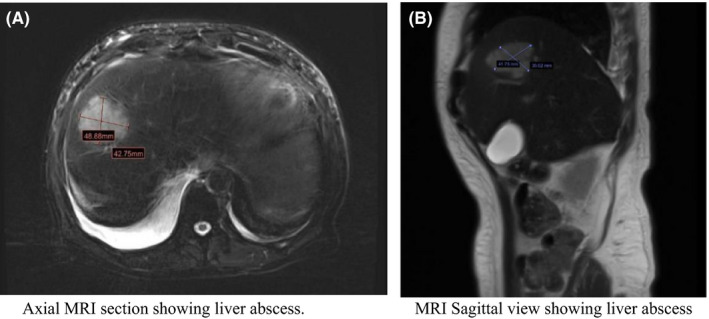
(A) Axial MRI section showing liver abscess. (B) MRI Sagittal view showing liver abscess
